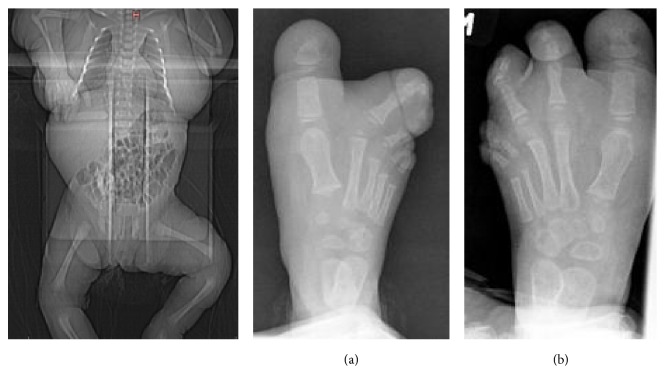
X-ray. (a) Right foot; (b) left foot.
